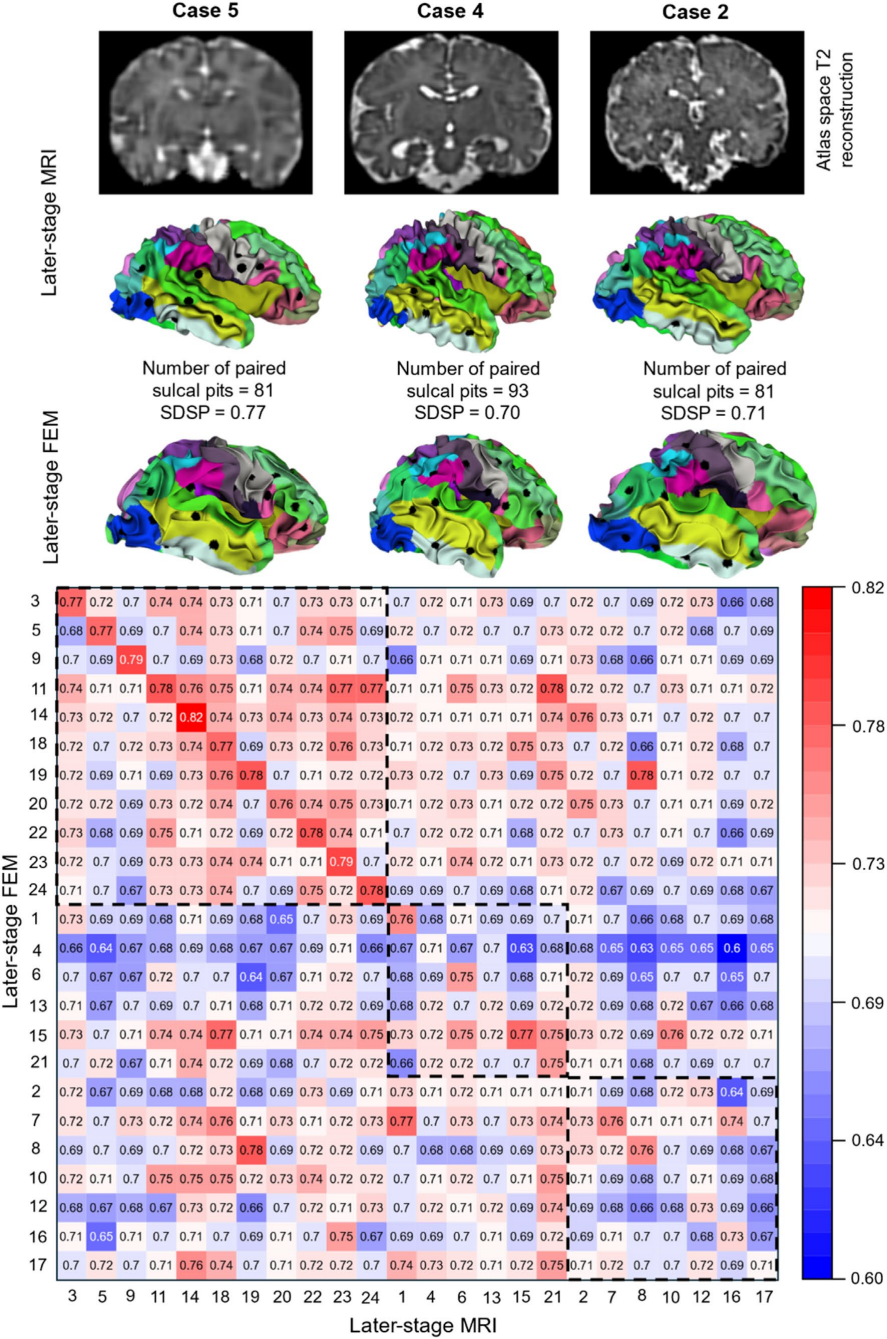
Sulcal pits‐based similarity (SDSP) between later‐stage FEM models and later‐stage MRI scans across all subject pairs. Diagonal values indicate within‐subject similarity, while off‐diagonal values represent between‐subject similarity. Rows 1–11 (top‐left dashed box): Cases showing higher SDSP values between the later‐stage FEM model and their corresponding later‐stage MRI scans compared to unrelated cases. Case 5 is presented as a representative example, including extracted sulcal pits from the FEM model and MRI scans, along with a coronal view of the T_2_‐weighted MR image in atlas space. Rows 12–17 (middle dashed box): Cases displaying higher similarity between the later‐stage FEM model and MRI scans from the same fetuses, even though the greatest similarity was not observed between early‐stage and later‐stage MRI scans. Case 4 is shown as an example, including sulcal pits and its MR image. Rows 18–24 (bottom‐right dashed box): Cases where the highest brain similarity is neither between early‐stage and later‐stage MRI scans nor between FEM models and their matching MRI scans. Case 2 is included as a representative example, along with its sulcal pits and MR image. Full orthogonal MRI views (coronal, axial, and sagittal) for these cases are provided in Figure S4. Different colors represent distinct cortical regions, with the full color legend provided in Figure S3.
